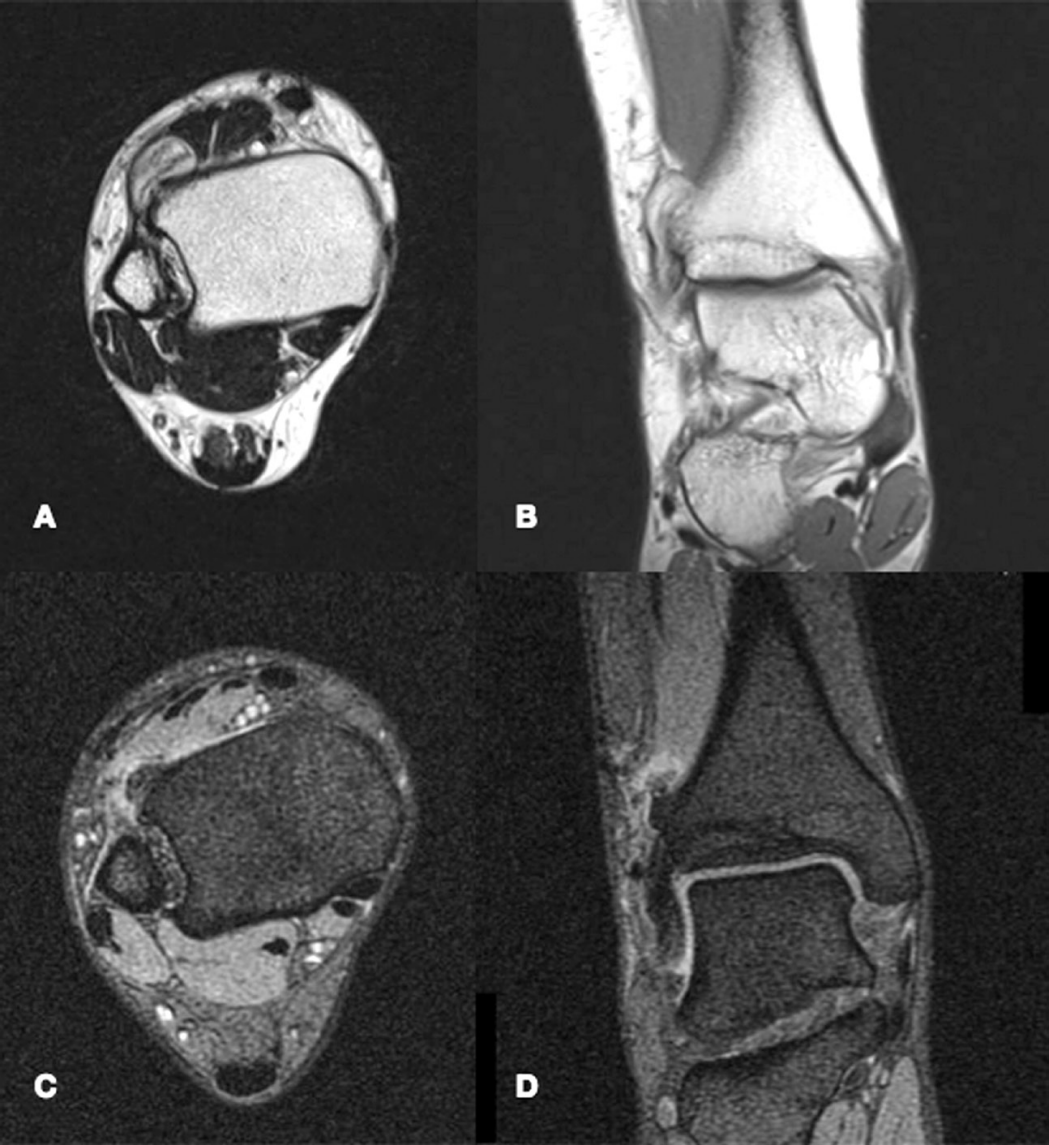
MRI images of the same lesion already pictured in Figure CT, displayed both with T1 weighted (A and B) and T2 weighted (C and D) sequences.
